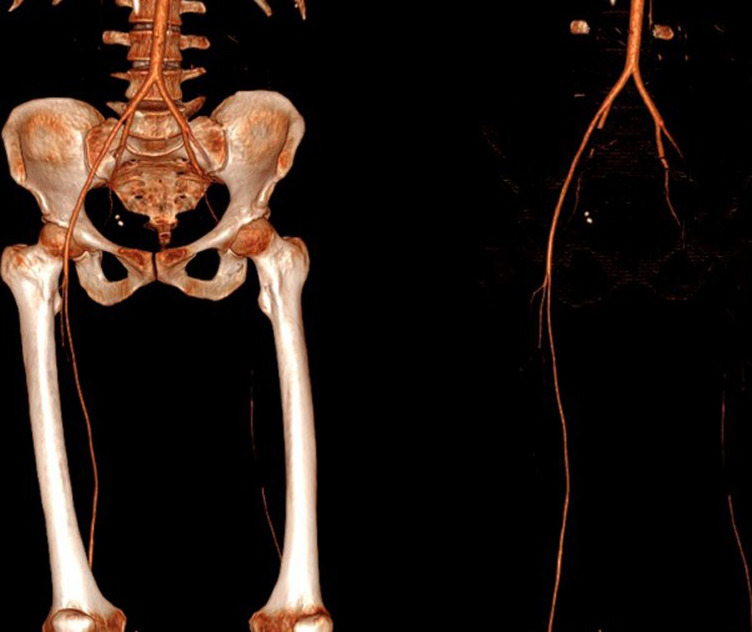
angio-CT scan of lower limb showing an interruption of vascular bed at the level of common femoral artery with partial resumption in the popliteal artery
